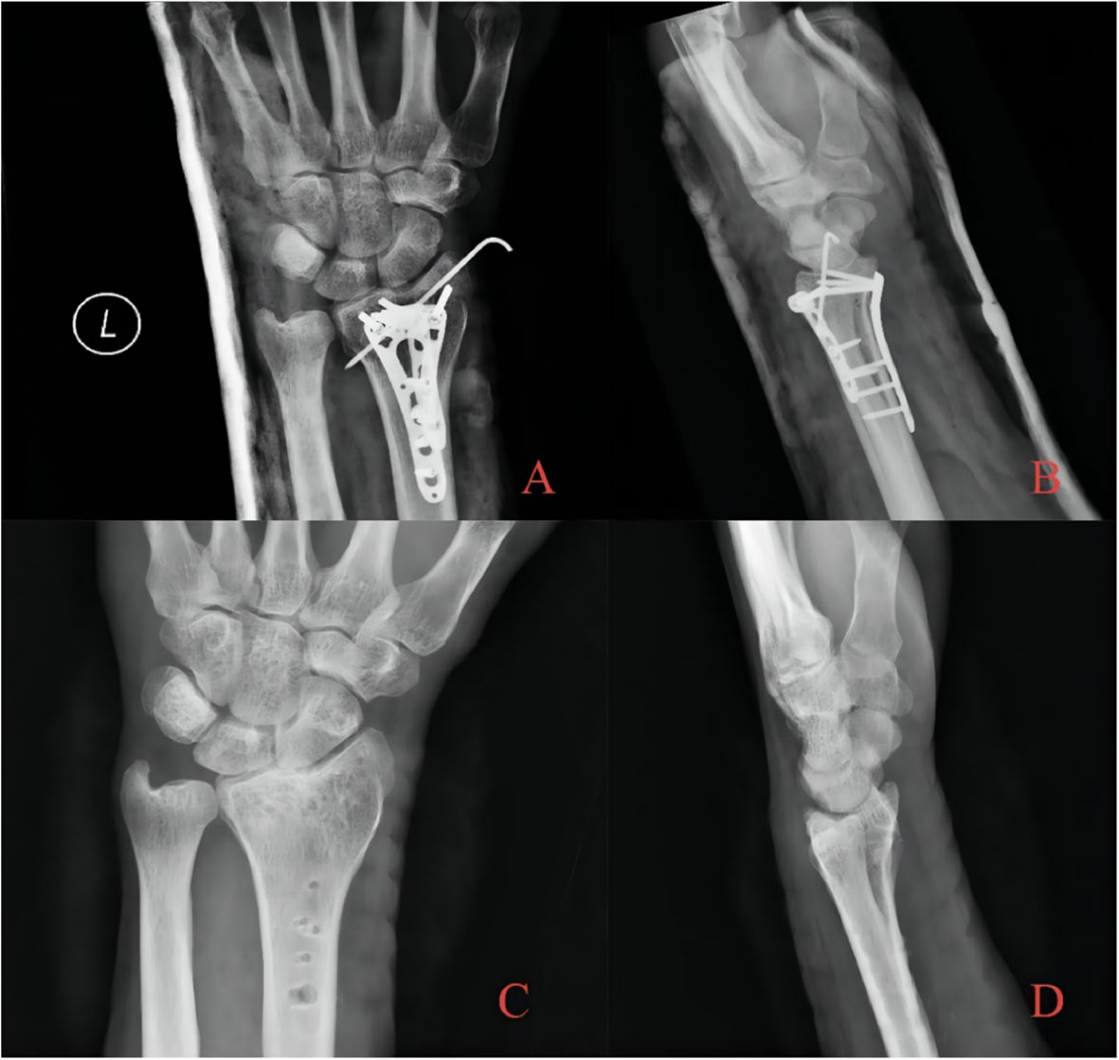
After satisfactory reduction, locking plates were used on both the dorsal and palmar sides. Kirschner wires for auxiliary fixation were retained for 8 weeks and were removed afterwards. The surgical results were good. The long-term follow-up after 30 months showed that the height of the articular surface was well maintained, and there was no obvious collapse of the articular surface. The internal fixator was removed for patient A Immediate postoperative AP radiography; B Immediate postoperative lateral radiography; C AP radiography after removal of internal fixation with follow-up over 30 months. Radial height was well maintained, and the articular surface was congruent in general; hence, no obvious articular step-off developed. D Lateral radiography after removal of internal fixation
